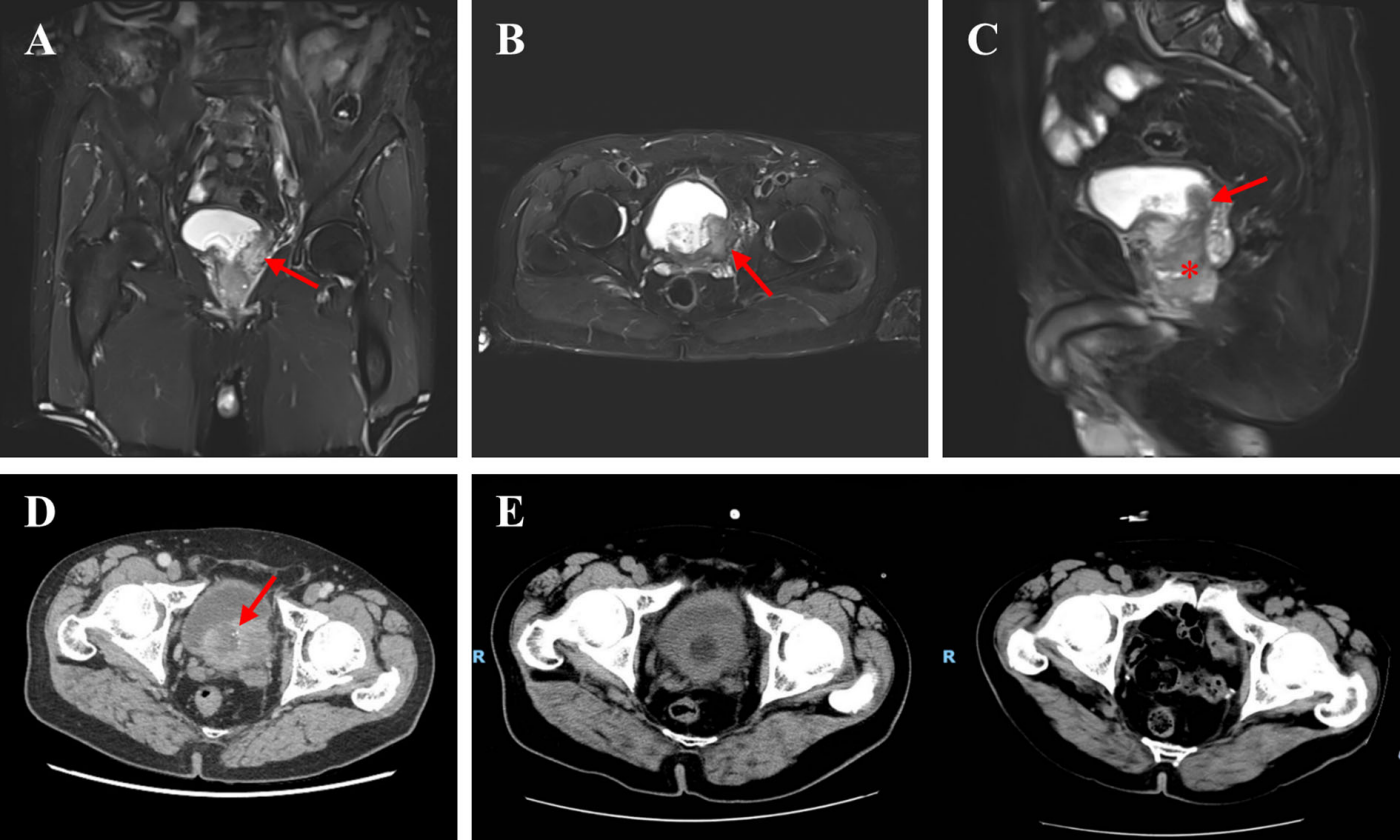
Preoperative and postoperative imaging of bladder mucinous adenocarcinoma. (A–C) T2-weighted pelvic MRI demonstrate an intravesical lesion (arrows) arising from the left posterior bladder wall in the coronal (A) axial (B) and sagittal (C) planes. The asterisk (*) in (C) indicates tumor invasion into the prostate. (D) CT urography reveals a posterior bladder wall mass with heterogeneous hypodensity (arrowheads), suggesting mucin deposition. (E) Postoperative CT at 6 months after radical cystectomy with pelvic lymphadenectomy demonstrates no pelvic fluid, bowel obstruction, or metastatic recurrence. CT, computed tomography; MRI, magnetic resonance imaging.
